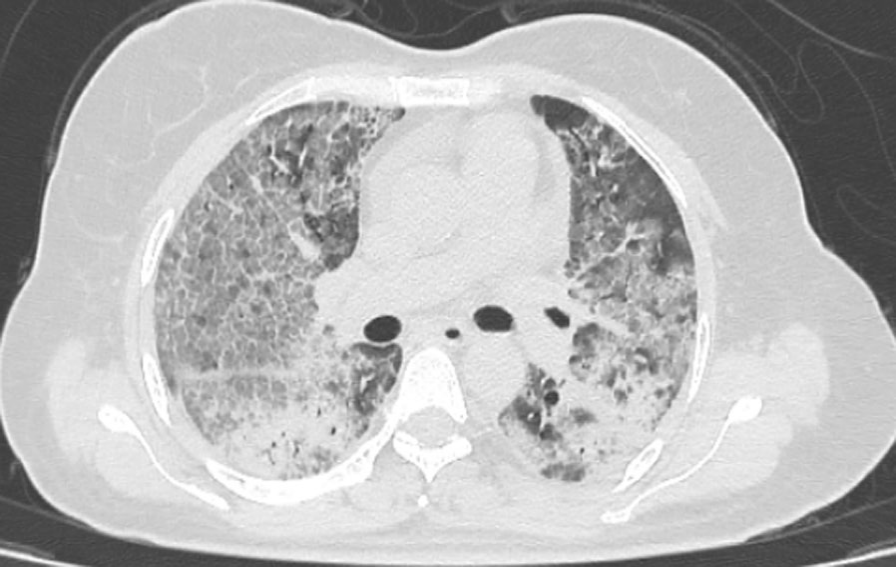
Axial CT image indicating severe disease severity with ARDS pattern (CTSS 25). Axial CT image shows diffuse extensive bilateral GGOs with crazy paving and multifocal consolidation bilaterally
